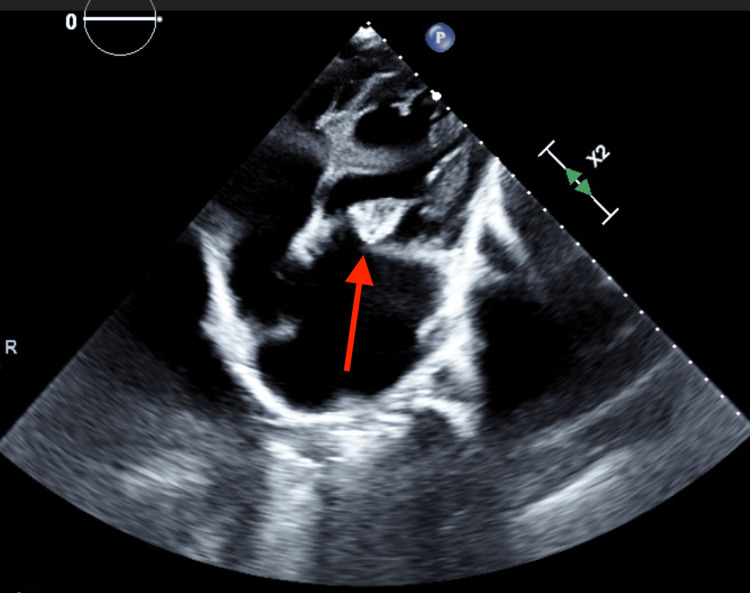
TTE The red arrow indicates mitral valve vegetation TTE: transthoracic echocardiogram
